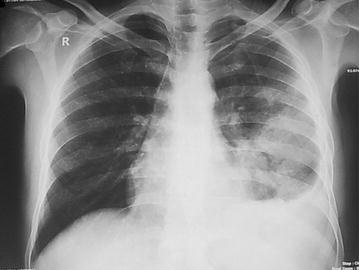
Chest x-ray posterior–anterior view after 2 weeks of treatment showing radiological improvement
